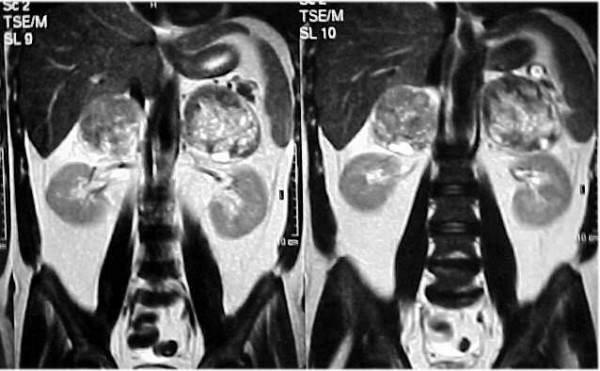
T_2 _weighted MR image showing disseminated foci of high heterogeneous signal intensity.
